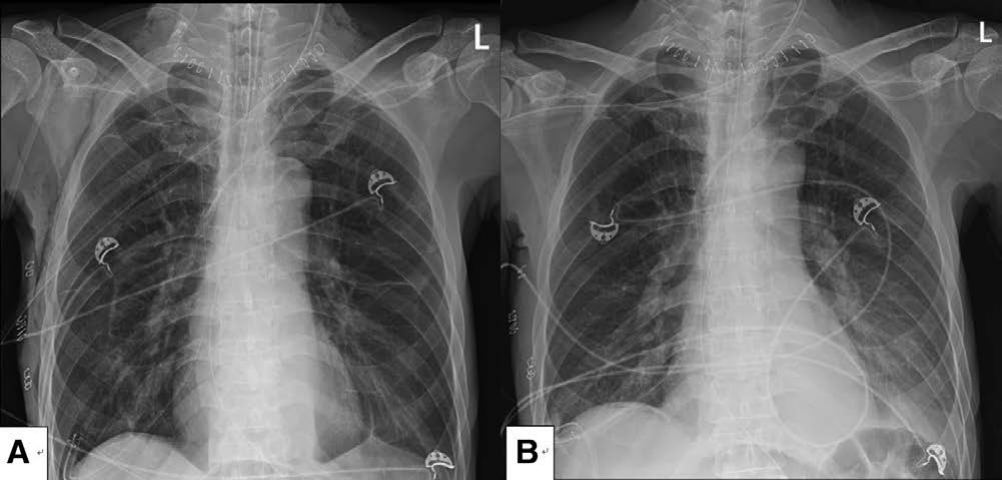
Postoperative chest radiography. A. Capnomediastinum on postoperative day 0. B. Extubation was performed on postoperative day 2.
